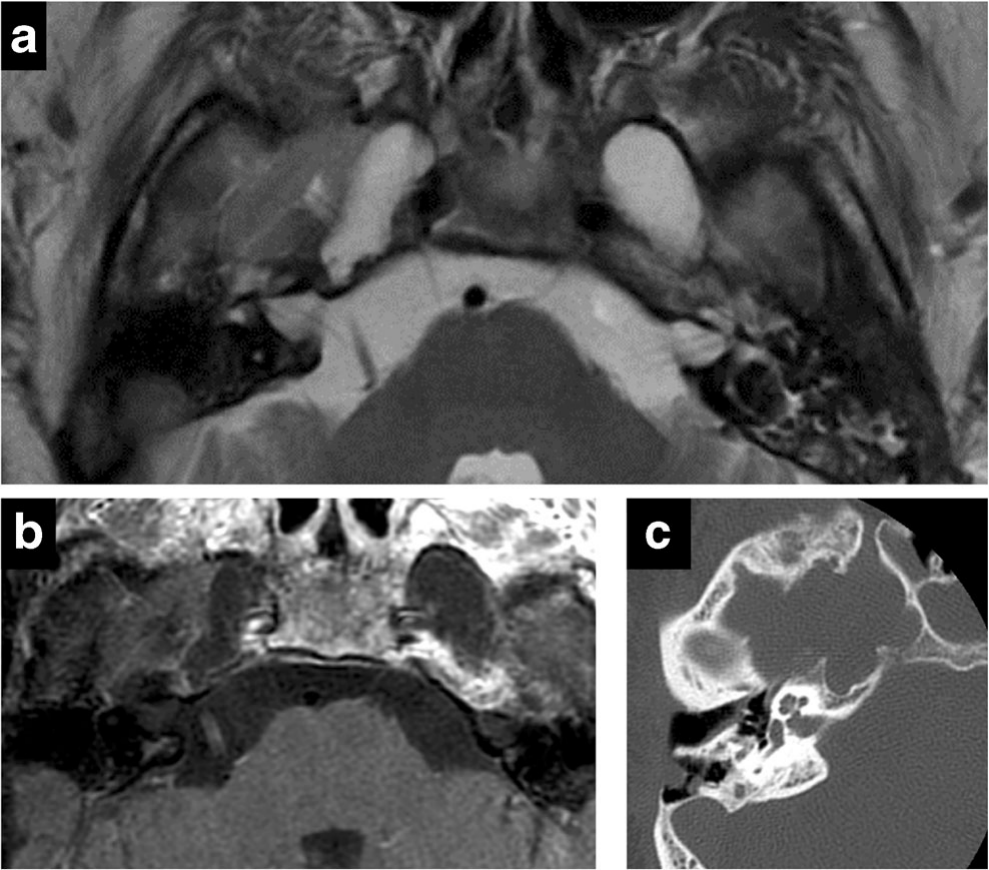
Dural ectasia in neurofibromatosis type 1. Axial T2 (a), axial C+ (b), CT (c). Bilateral enlargement of Meckel’s cave—CSF isointense and no abnormal enhancement. Smooth scalloping and remodelling of petrous apex on CT (c)
